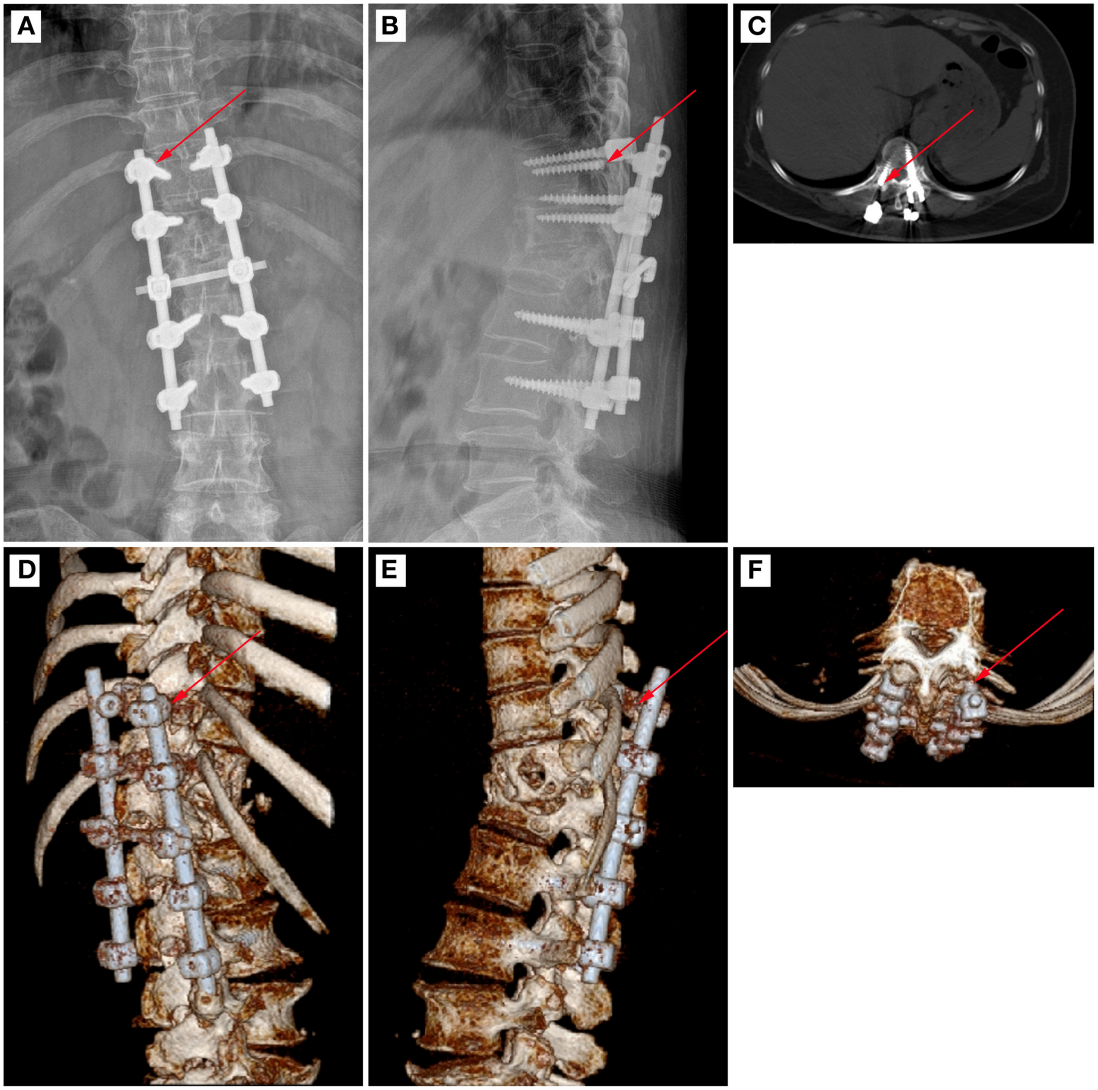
A typical case of pedicle screw fracture after spinal tuberculosis surgery. The arrow marks the location of pedicle screw fracture. (A) Post-operative X-ray image in the posterior–anterior position. (B) Post-operative X-ray image in the lateral position. (C) Post-operative CT image in the cross-section. (D) Post-operative CT reconstruction image in the coronal position. (E) Post-operative CT reconstruction image in the sagittal position. (F) Post-operative CT reconstruction image in the cross-section.
